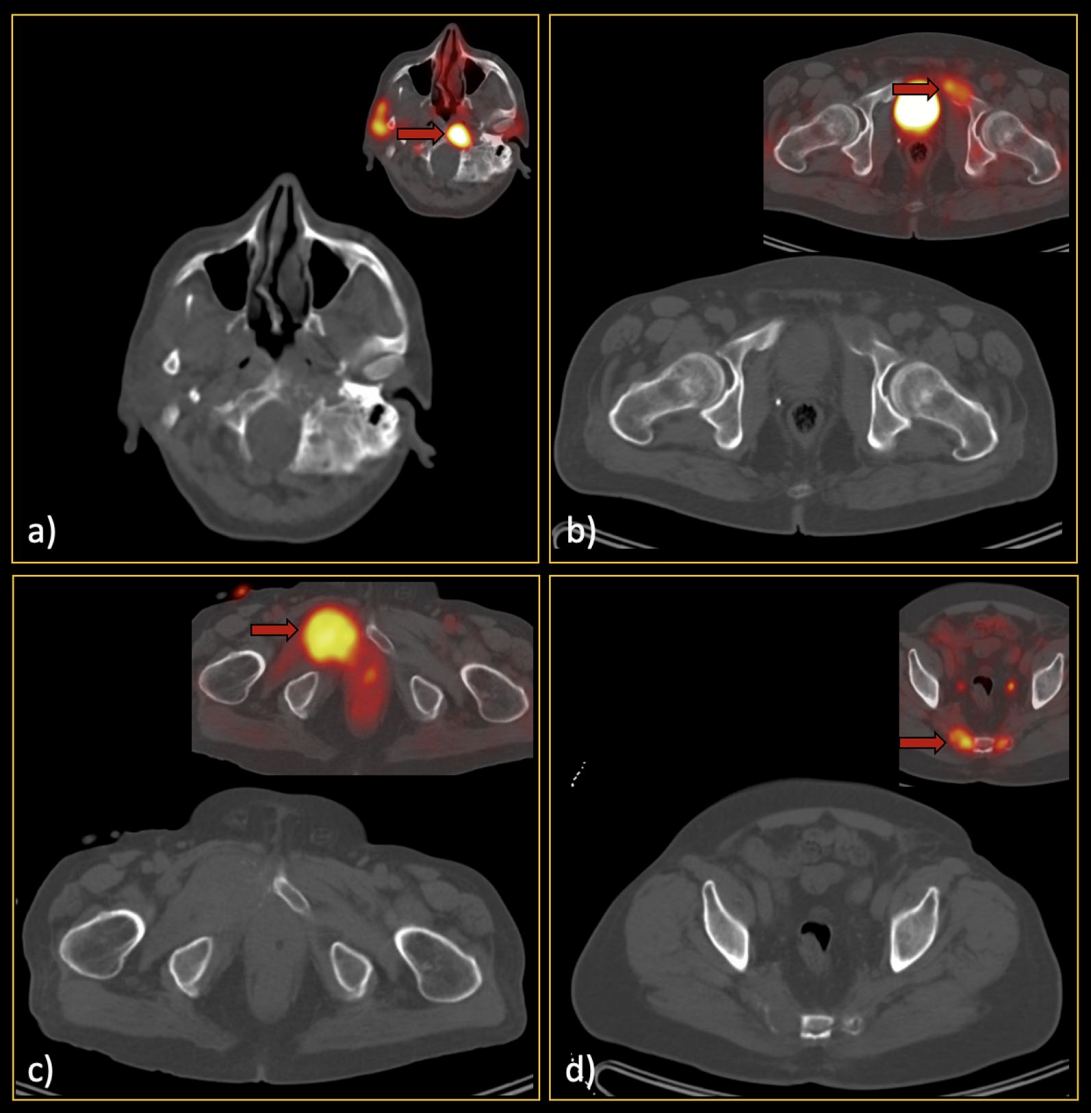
Examples of the typical bone metastases (a–d) with the detected prominent features, including soft tissue component, ill-defined border, cortical destruction, and maximal transverse diameter of more than one centimetre. Locations of the metastases are shown with red arrows on the hybrid ^68^Ga-PSMA PET/CT images
